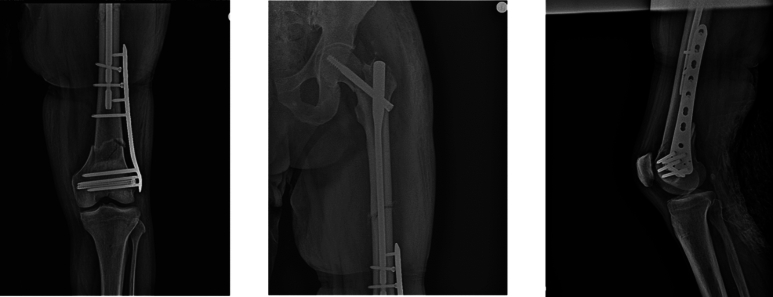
Immediate post-operative control X-ray.
